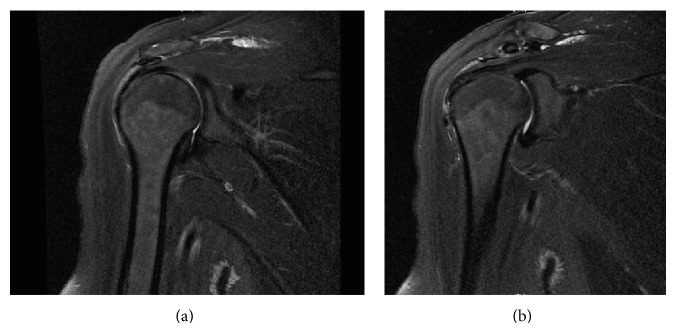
Preoperative T2-weighted, coronal oblique MRI showing (a) a small, nearly full-thickness tear of anterior supraspinatus tendon as well as (b) multiple extratendinous ossific densities consistent with synovial chondromatosis.
